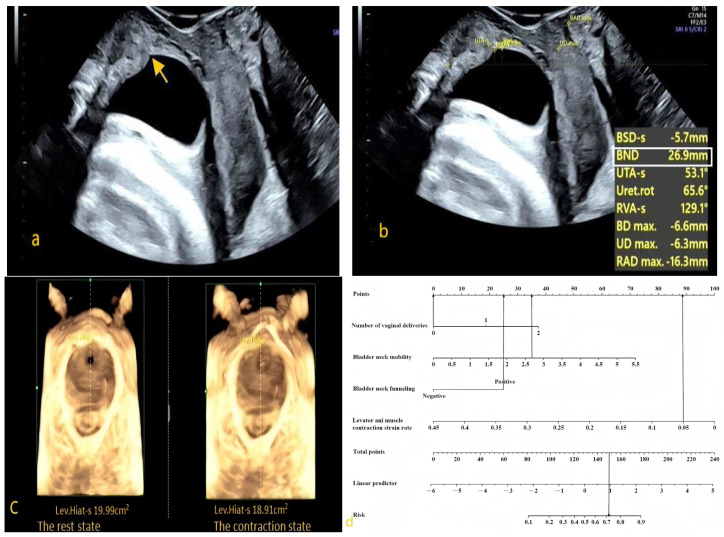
Example of the application of the nomogram. (a) Bladder neck funneling under the Valsalva maneuver (yellow arrow); (b) Bladder neck mobility (white rectangular box); (c) Puborectal muscle hiatus area under the resting and pelvic floor muscle contraction states; (d) Pelvic floor ultrasound examination showing bladder neck funneling (25 points), bladder neck mobility of 2.69 cm (35 points), and puborectal muscle contraction strain rate of 0.05 (89 points). The total score is approximately 149 points (combining 25 + 0 + 35 + 89), predicting a 71.2% probability of stress urinary incontinence for this patient.
